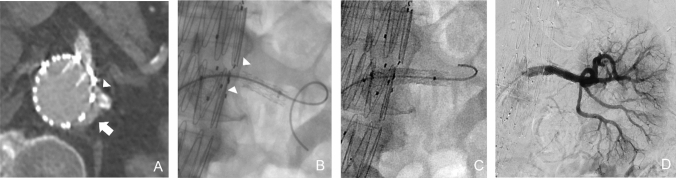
Type III EL in a F-EVAR. (A) Axial CT image shows junctional separation between the main body and fenestrated left renal stent (arrowhead) resulting in type III EL (arrow). (B) Fluoroscopic image confirms a visible gap between the two stent components (arrow heads). (C) Additional renal stent graft deployed to bridge the gap. (D) Angiogram shows no residual EL
